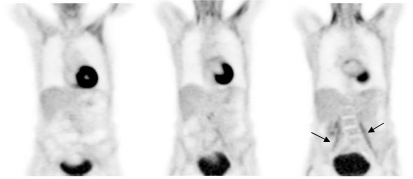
FDG PET scan (coronal view).There is increased activity seen in the neck and shoulder muscles. Moderate uptake is also seen in the psoas muscles in this patient with scoliosis (arrows) [Image courtesy of Centre for Molecular Imaging, Peter MacCallum Cancer Centre, East Melbourne, Victoria, Australia].
